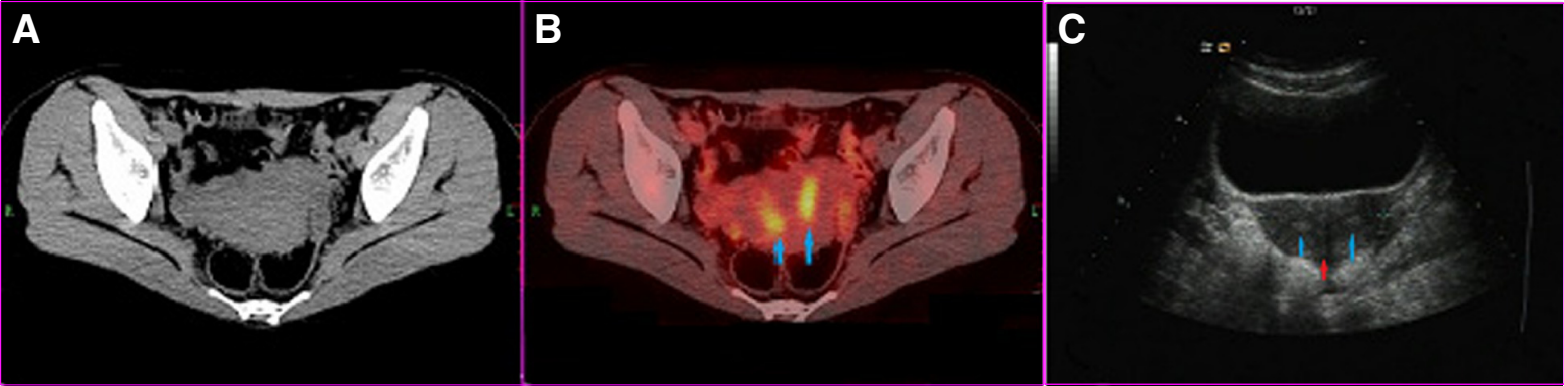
Two linear foci of FDG accumulation in the central part of the uterus were found in the PET/CT fusion image (B, blue arrow). Ultrasound scan showed two cavities (blue arrows) with muscular septum (red arrow) (C).
